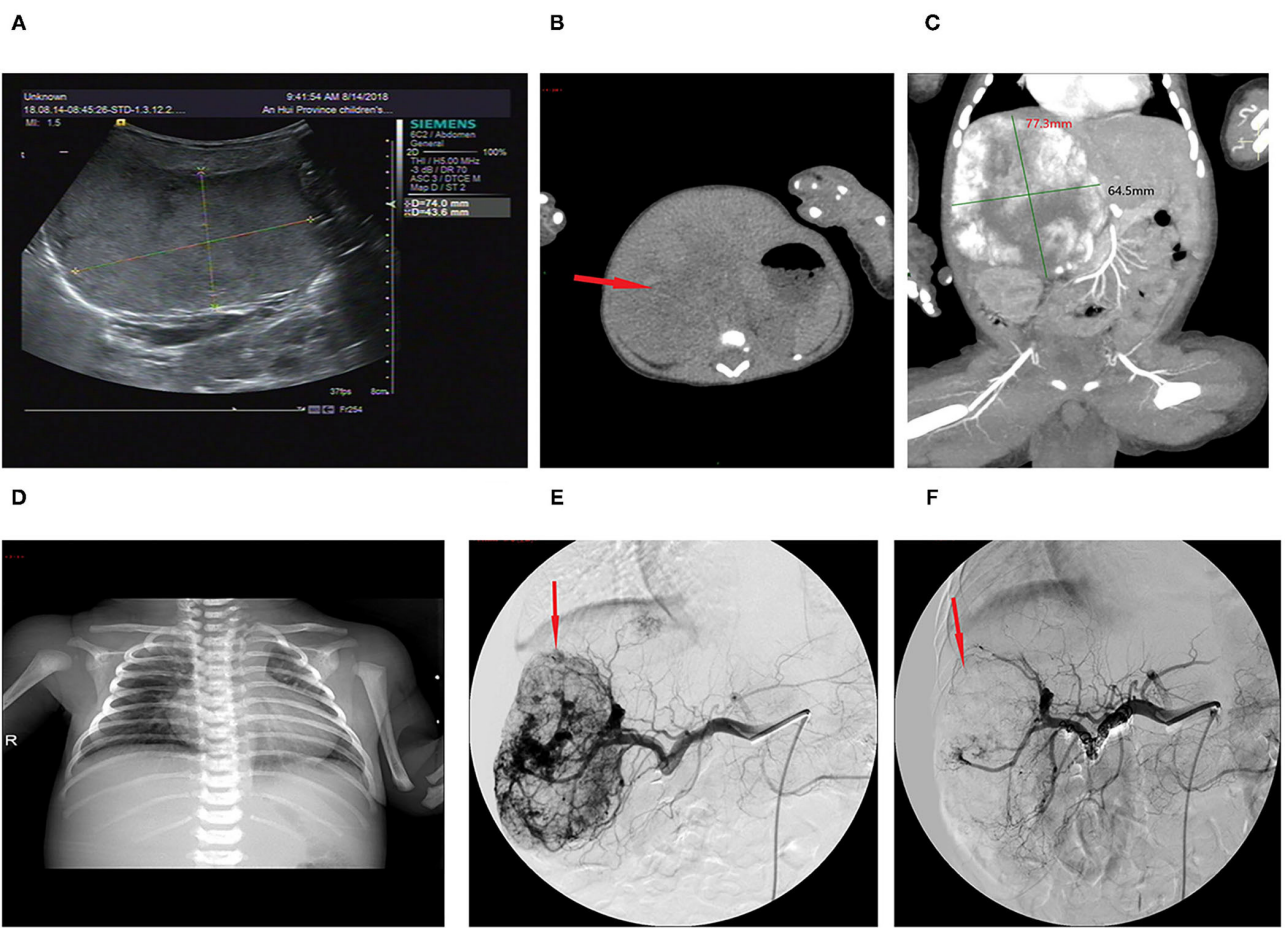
Imaging of the hepatic hemangioma for case 6. (A) Ultrasonography showed a hyperechoic solid lesion in the liver. (B) CT showed that the lesion was a large soft tissue mass. (C) Contrast-enhanced CT showed obvious arterial phase enhancement inside the mass. (D) CXR showed enlargement of the heart shadow. (E) CTA showed an abundant tumor blood supply before embolization. (F) After TAE, more than 80% of the tumor-feeding arteries were embolized. TAE, transarterial embolization; CTA, computed tomography–angiography; CXR, chest X-ray. The red arrow indicates the lesion.
